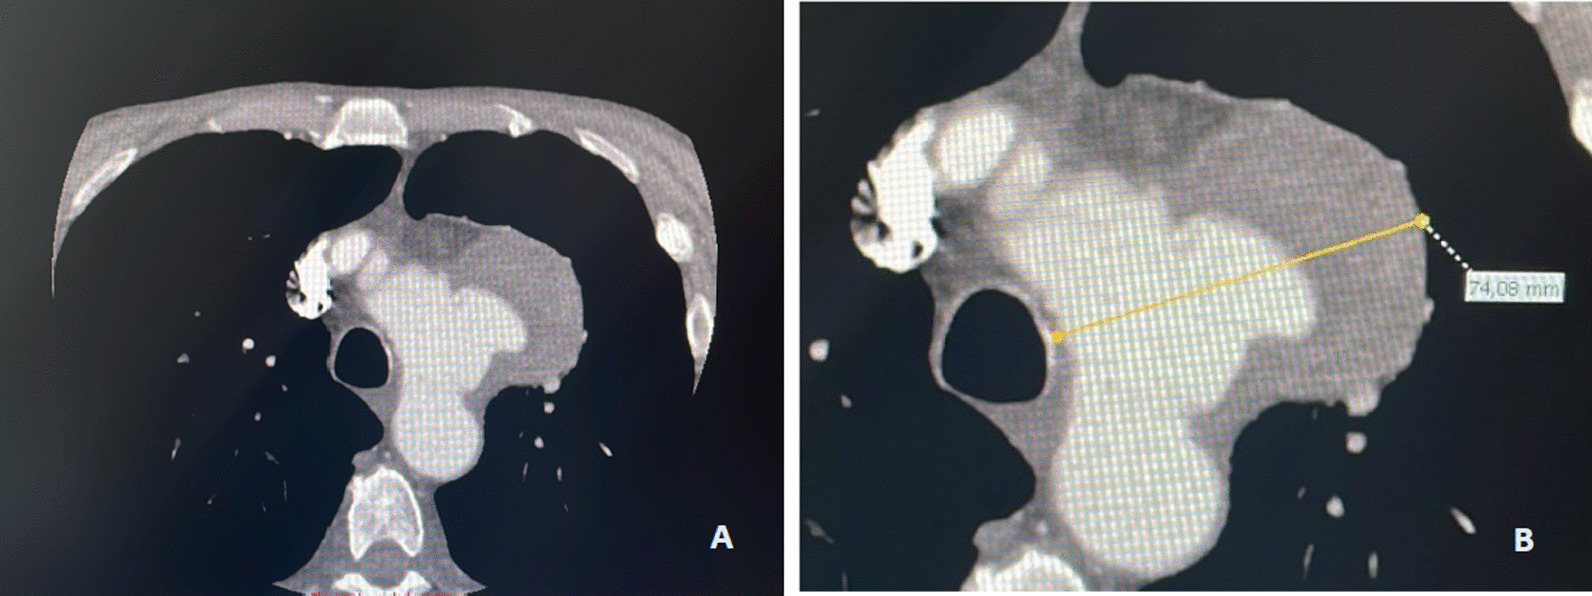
Pre-treatment figures from CTA showing aneurysm. A more detailed description in the text
